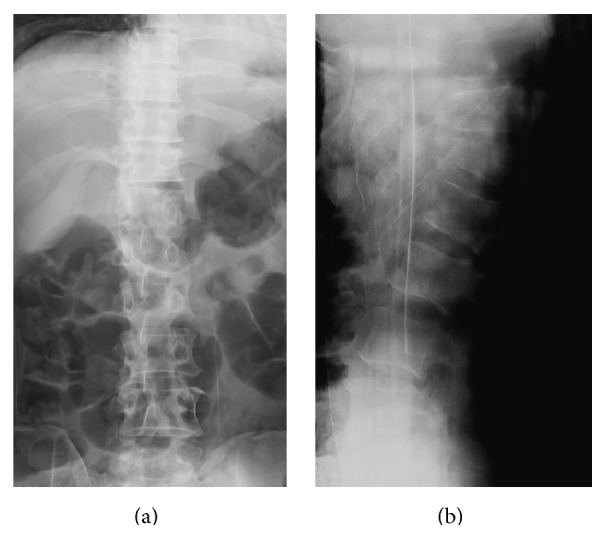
Preoperative radiographs. (a) Anteroposterior (AP) view, (b) lateral view. Fracture and wedge deformity of the 12th thoracic (T12) vertebra.
